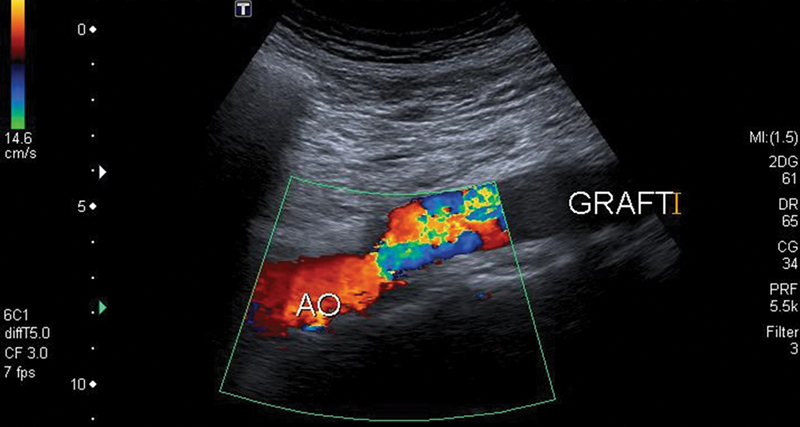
Color-flow Doppler image of the proximal anastomosis.
